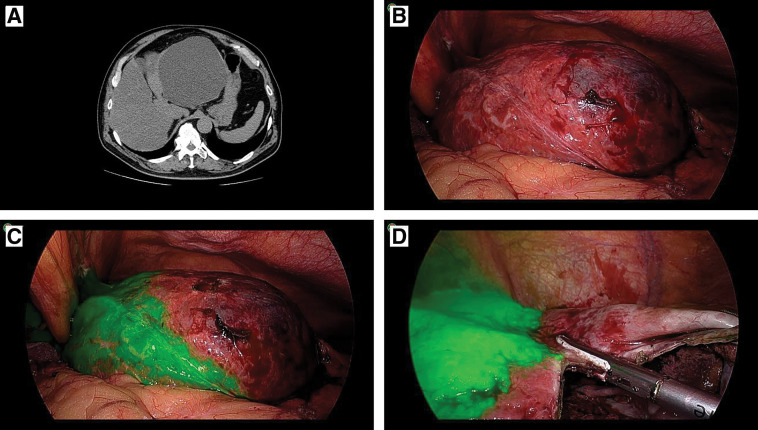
Preoperative CT and intraoperative findings of case 2. (A) Abdominal CT reveals a cystic lesion in the lateral segment of the liver, with a thin layer of parenchyma covering the cyst. (B) The boundary between the parenchyma and the cyst wall is not clearly visible under normal light. (C) Full-color overlay mode clearly delineates the appropriate line for deroofing (ICG-positive liver parenchyma is shown in green). (D) Surgery is conducted safely using the full-color overlay mode. ICG, indocyanine green
